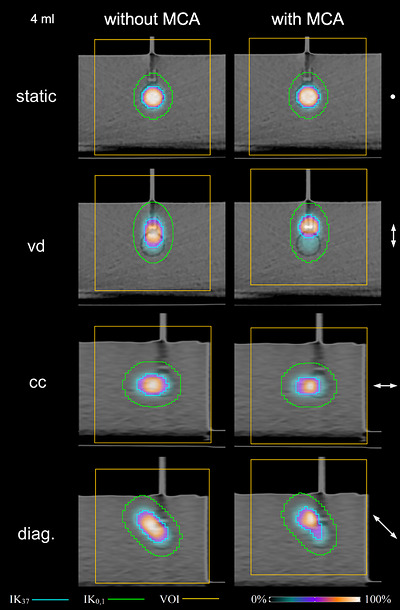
Sagittal PET/CT fusion images of the 4‐ml sphere exemplary for used motion conditions, shown without motion correction (MCA) on the left and with MCA on the right. Rows correspond to the four motion states: static (no motion), ventrodorsal motion (vd), craniocaudal motion (cc), and diagonal motion (diag.). Motion causes characteristic elongation of the activity distribution along the corresponding trajectory, resulting in direction‐dependent shape deformation.
